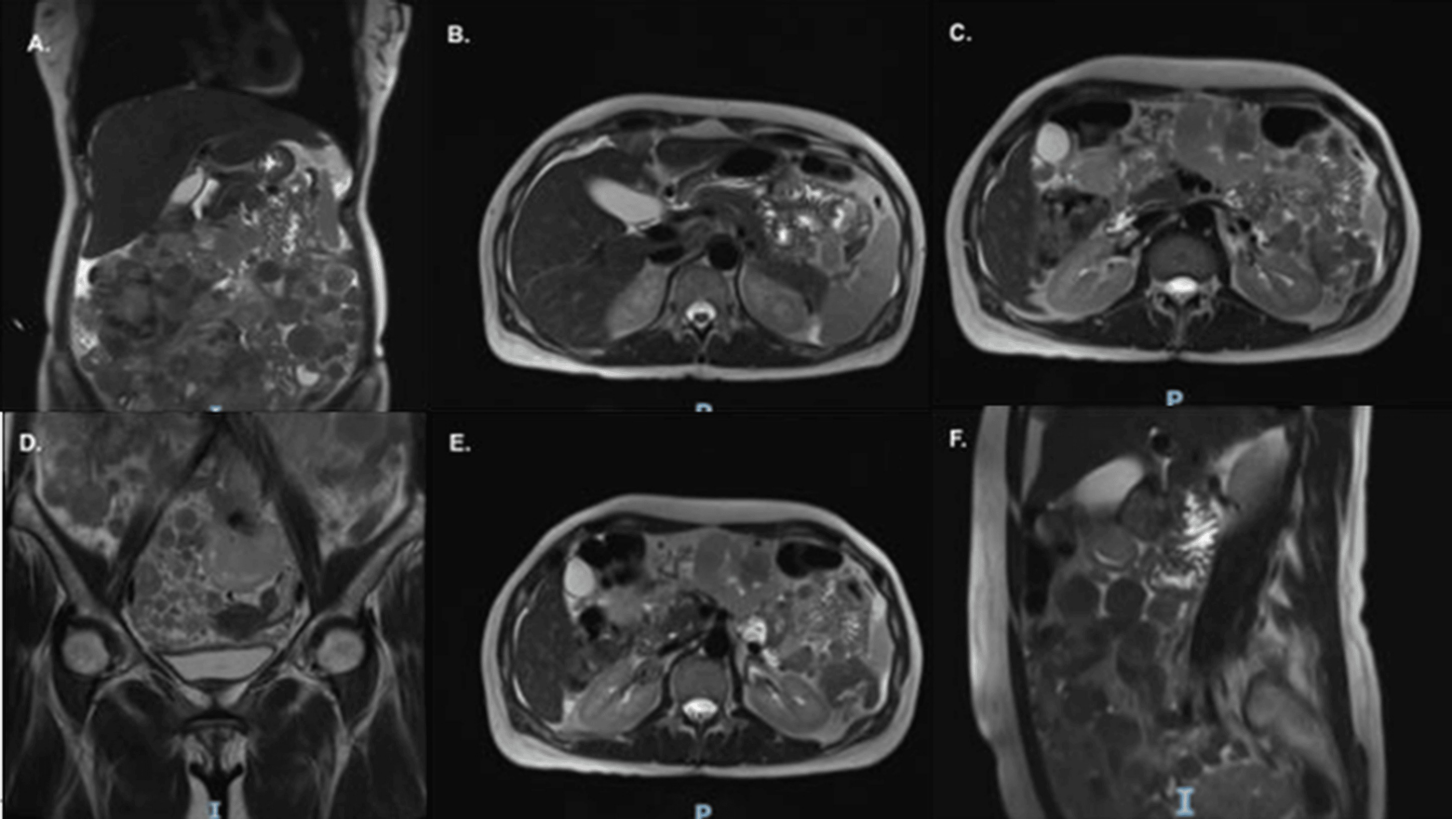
Pre-treatment abdominopelvic MRI (T2-weighted sequences) demonstrating extensive peritoneal sarcomatosis. (A) Coronal view showing diffuse hyperintense peritoneal implants. (B, C, F) Axial and sagittal views confirming widespread peritoneal disease with necrotic components. (D) Coronal T2-weighted image showing pelvic extension of hyperintense peritoneal implants with mild bowel compression. (E) Axial T2-weighted image demonstrating multiple inframesocolic hyperintense peritoneal masses with central necrotic areas.
